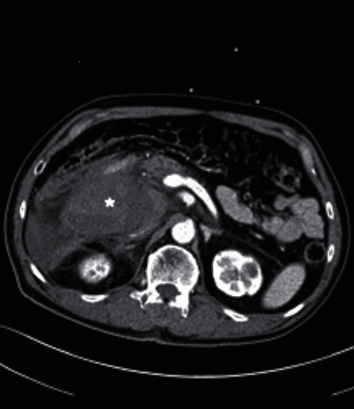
Axial CT image showing a large acute retroperitoneal hematoma (asterisk) centered around the paraduodenal region extending anterior to the right anterior pararenal fascia.
